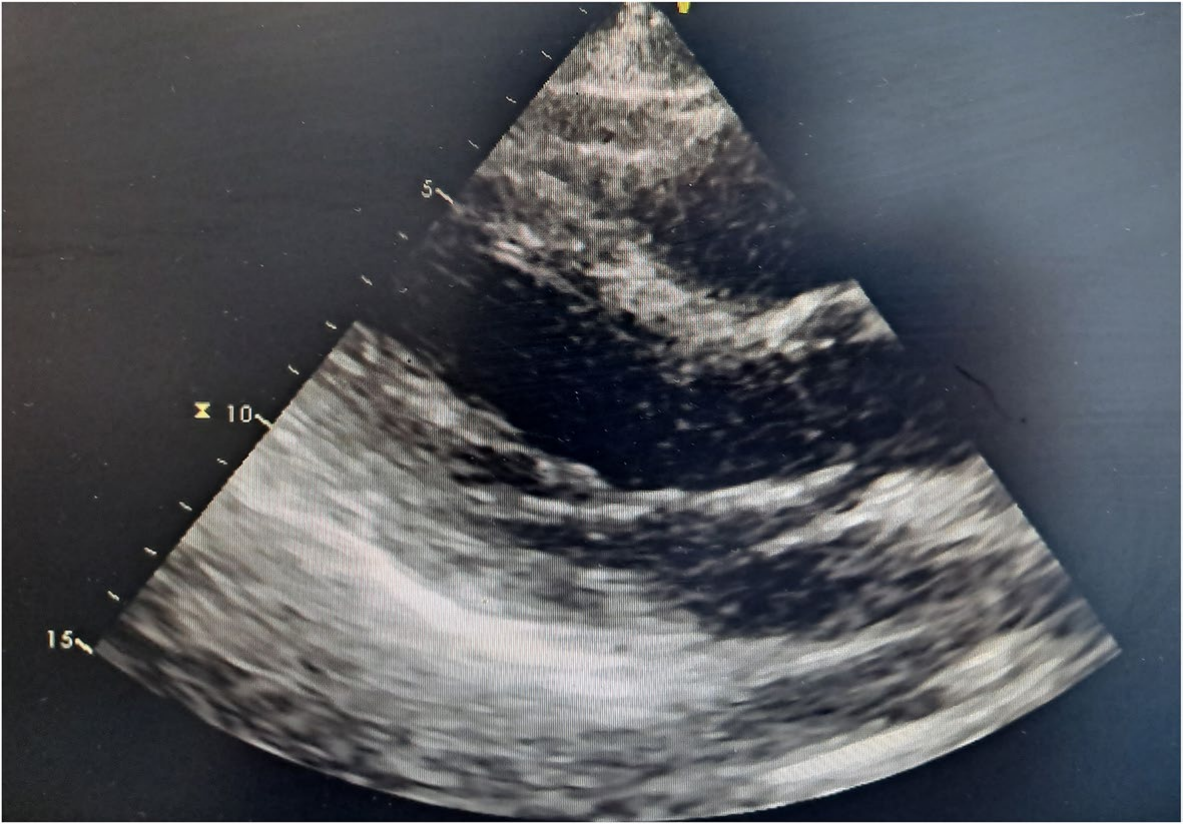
The echocardiography after tislelizumab therapy in August 2021. Compared to that of April 2021, the left atrial mass vanished
